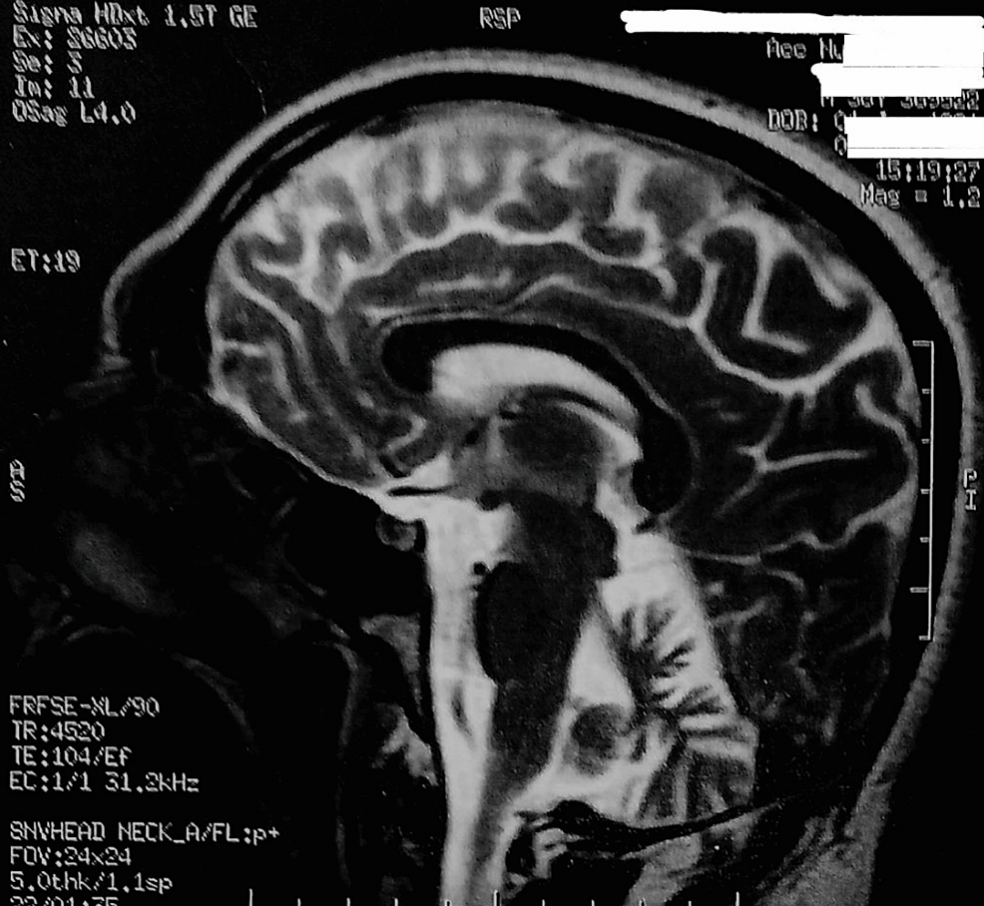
T2-weighted sagittal MRI showing moderate cerebellum atrophy.
